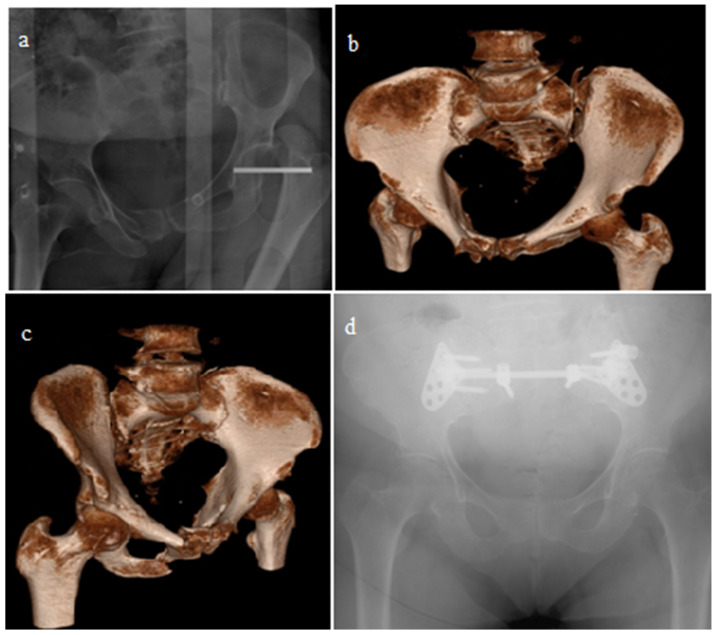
A 37-year-old male patient with bilateral sacroiliac fracture dislocation. Pelvic radiography (a) and computed tomography images (b,c) at the time of injury. Pelvis radiograph at the fifth year of patient follow-up (d). Fixation of sacroiliac joints by posterior approach with plate–screws.
